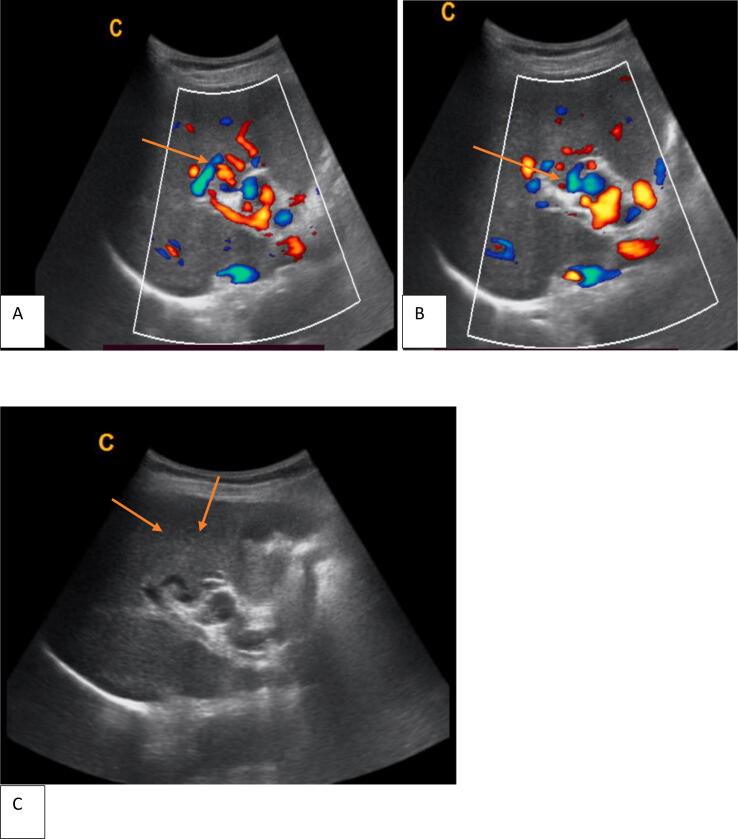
Transverse RUQ gray scale and color Doppler images show. The main portal vein cannot be identified, with the portal vein bed containing multiple clusters of serpiginously enhancing vessels extending into the liver (Orange arrows in panels A, B, AND C).
